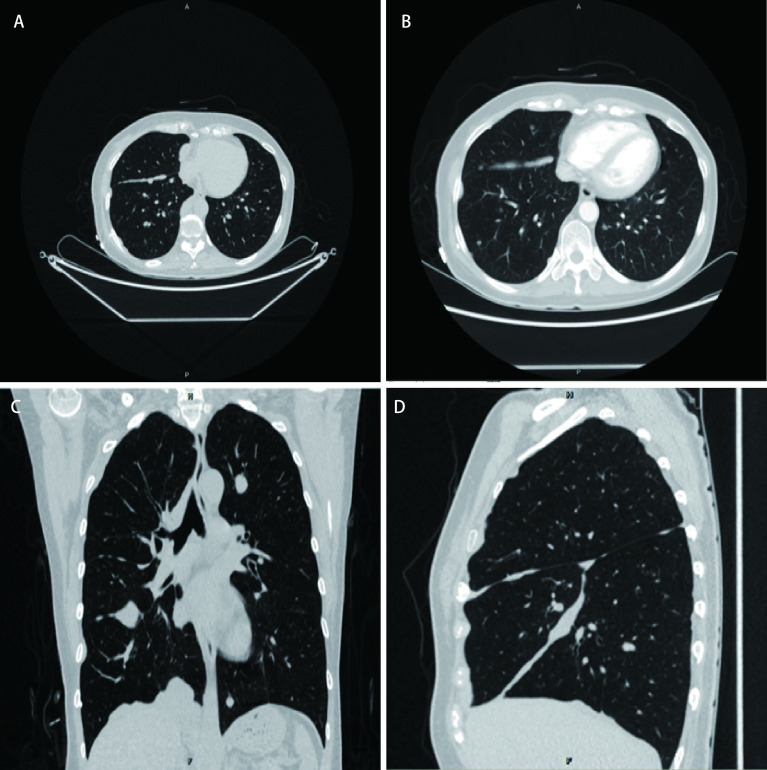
胸部CT平扫+增强示双肺及双侧叶间裂可见多发大小不等实性结节。A：胸部CT平扫右肺叶间裂可见多发大小不等实性结节影，右侧胸膜呈不均匀或结节状增厚；B：与A图同一截面增强后可见叶裂间结节明显强化，右侧胸膜不均匀增厚并强化；C：胸部CT平扫冠状位重建可见双肺多发结节影，纵隔多发肿大淋巴结；D：胸部CT平扫右肺矢状位重建可见右肺水平裂及斜裂间多发大小不等实性结节影，右侧胸膜呈不均匀或结节状增厚 Chest CT plain scan + enhancement shows multiple solid nodules with unequal sizes in bilateral lobes and bilaterally lobed lobes. A: multiple solid nodule shadows with unequal sizes in right lobed lobes and uneven or nodular thickening in right pleura on chest CT plain scan; B: obvious enhancement in the same cross section as A, and uneven thickening and enhancement in right pleura; C: plain scan in chest CT In the coronal reconstruction, multiple nodular shadows of both lungs and swollen lymph nodes of mediastinum can be seen; D: in the sagittal reconstruction of the right lung on plain CT scan of the chest, multiple solid nodular shadows of unequal size can be seen between the horizontal and oblique fissures of the right lung, and the right pleura is uneven or nodular thickening. CT: computed tomography
